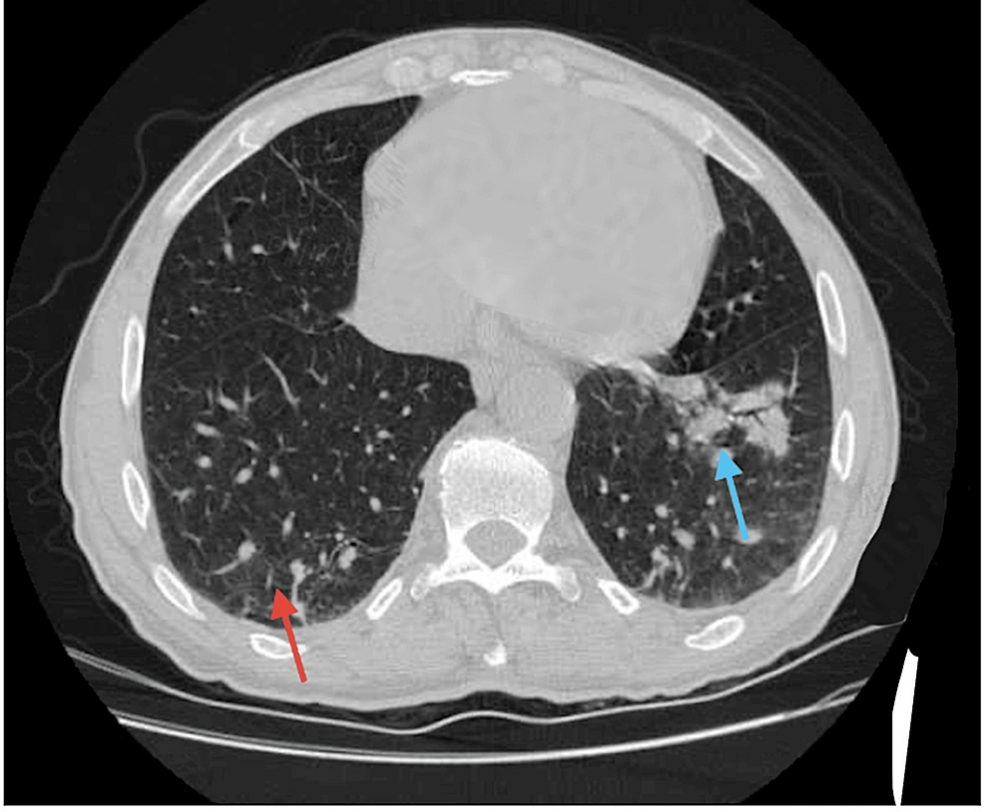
Computed tomography findings. The red arrow shows ground-glass opacities, and the blue arrow shows air bronchogram.
